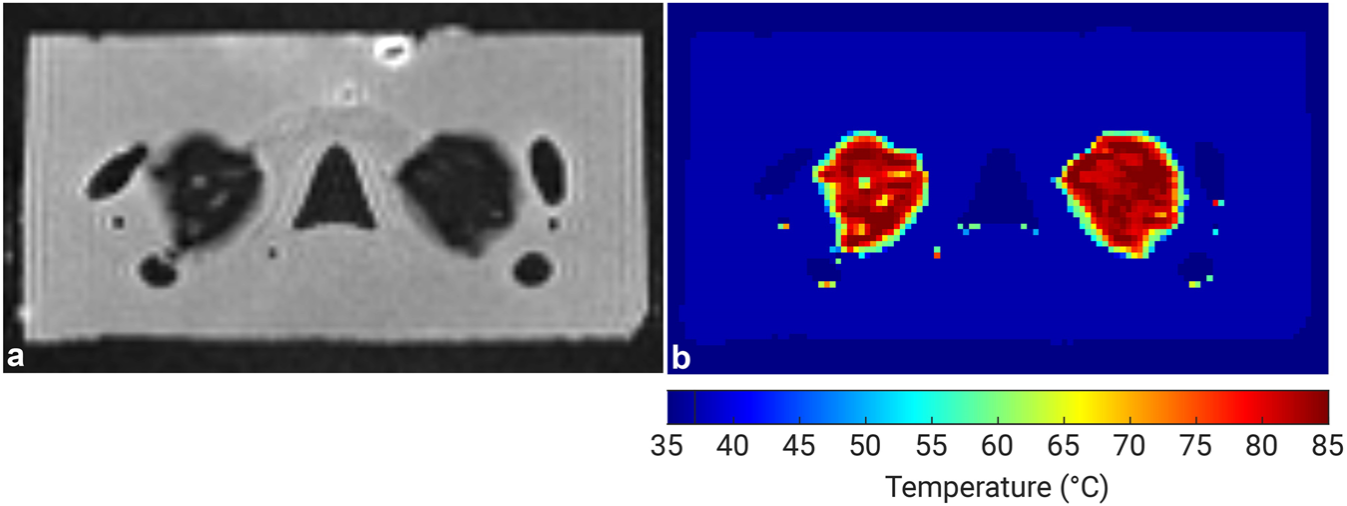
a Postablation T_2_-weighted magnetic resonance imaging scan (echo time = 400 ms) showing the lack of signal in the ablated areas. b The corresponding temperature map of the ablated areas
